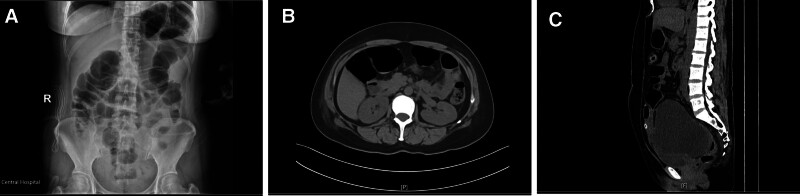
(A–C) Abdominal X-ray: Intestinal gas accumulation with atypical small fluid (A). Abdominal CT: intestinal dilation and gas accumulation (B). Abdominal and pelvic CT: pelvic encapsulated fluid accumulation (C).
